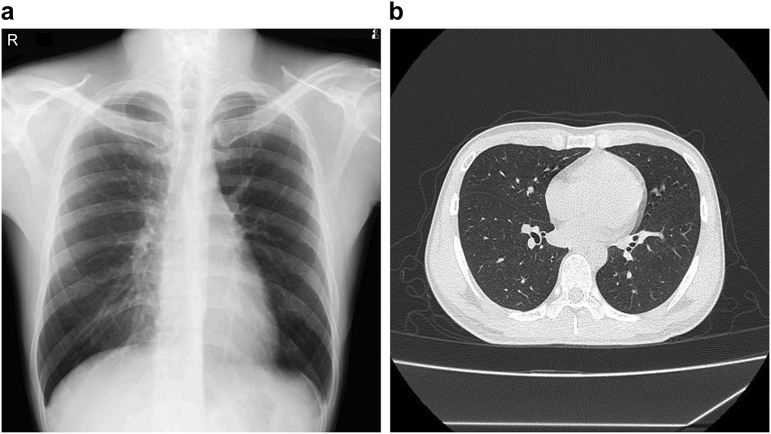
Chest X-ray and chest CT findings (A) Chest X-ray showed no abnormal shadows in the lung field. (B) Chest CT showed no pneumonia CT, computed tomography.
